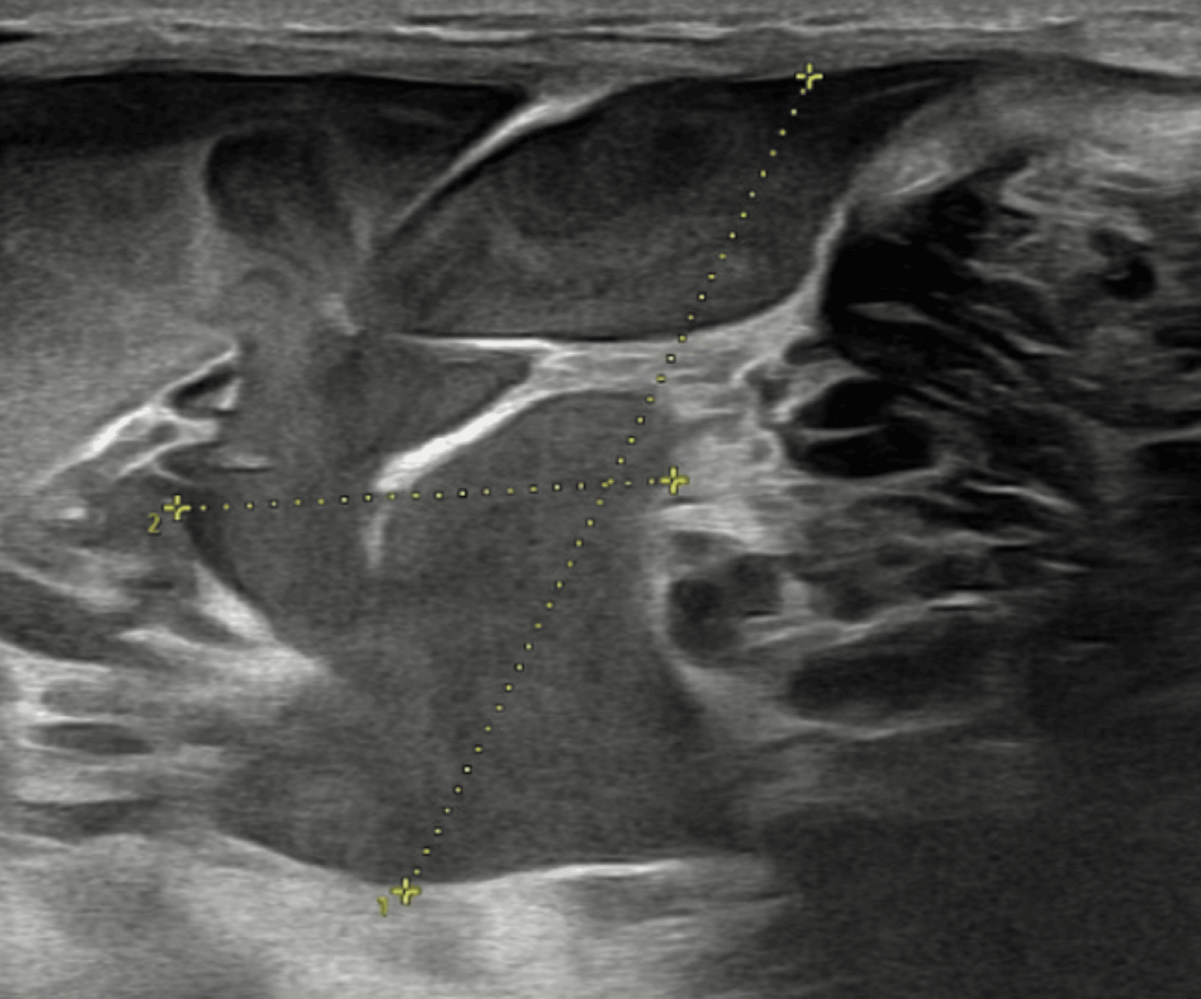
Ultrasonography of the right parotid region demonstrating a cystic lesion consistent with venolymphatic malformation.
